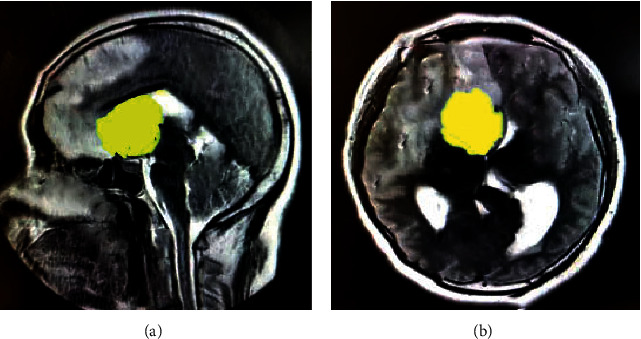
MRI images of brain tumor, where (a) is in the sagittal plane and (b) is in the coronal plane. The yellow part in the images indicated the tumor region.
